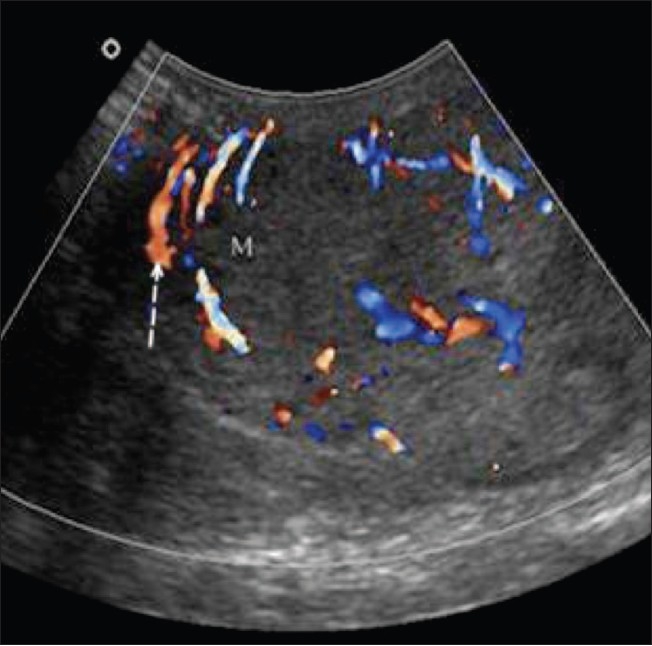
Non-seminomatous germ cell tumors. Color Doppler shows a focal lesion with increased vascularity (arrow)
